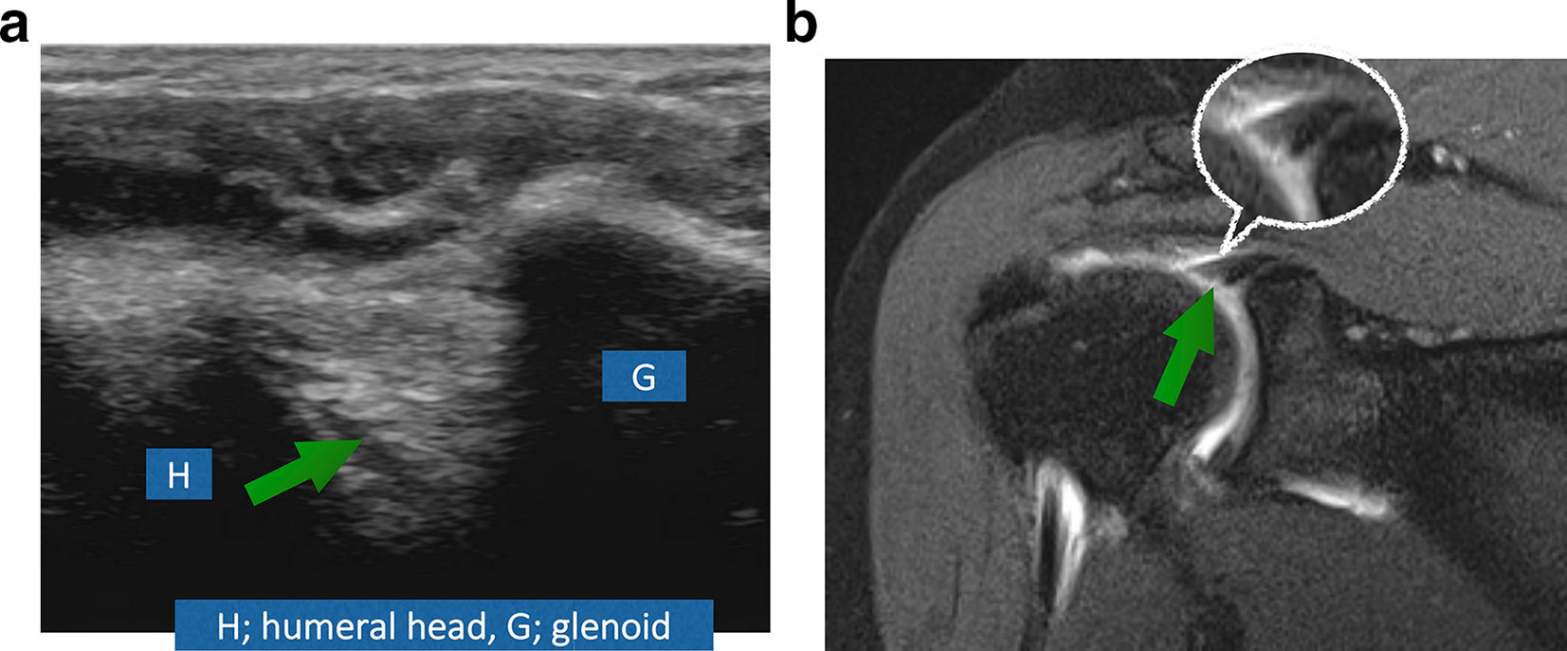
(A) A 22-year-old male patient with recurrent subluxations. Both ultrasound (Figure 4A) and MRI (Figure 4B) show fraying of the labrum in keeping with Type 1 SLAP lesion.
